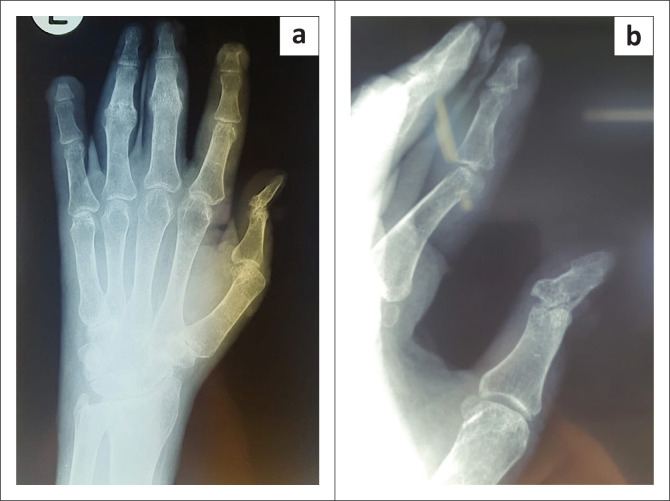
(a and b) X-ray of patient’s left hand, demonstrating osteoarticular destruction at multiple sites.
